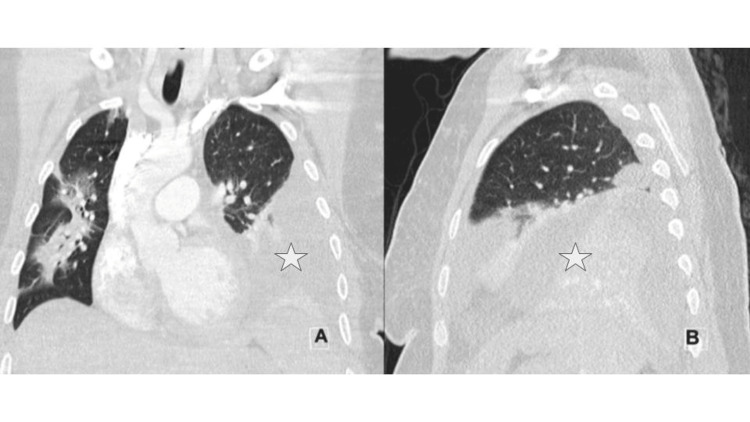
Computed tomography (CT) images showing a large left-sided empyema (white star) (A) Coronal view; (B) sagittal view
